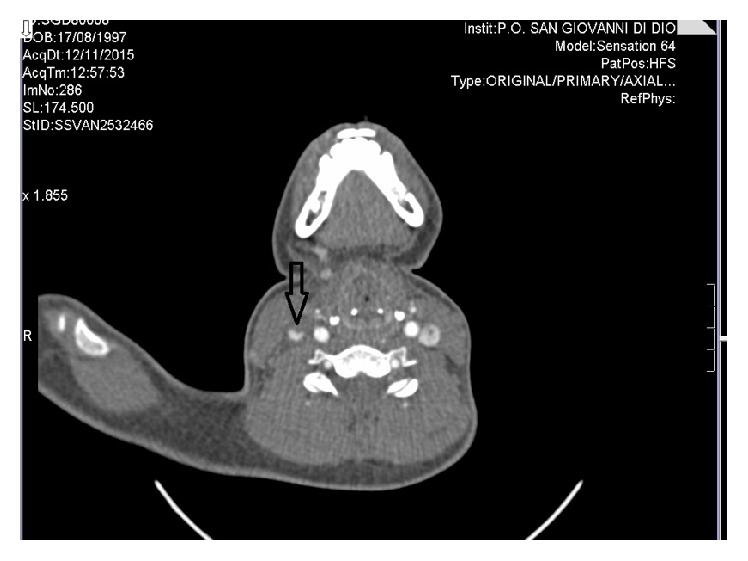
CT scan of the neck which shows (arrow) a filling defect of the right internal jugular vein due to partial thrombotic occlusion.
